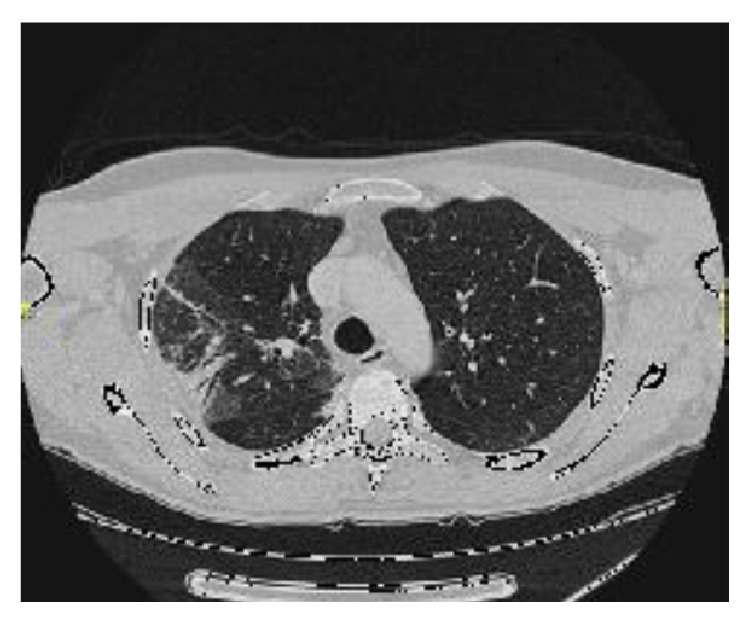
HR-CT lung pretreatment: interstitial consolidation with centrilobular branching linear structures with bronchial dilatation and multifocal areas of ground-glass attenuation at right superior lobe of lung in the posterior segment.
